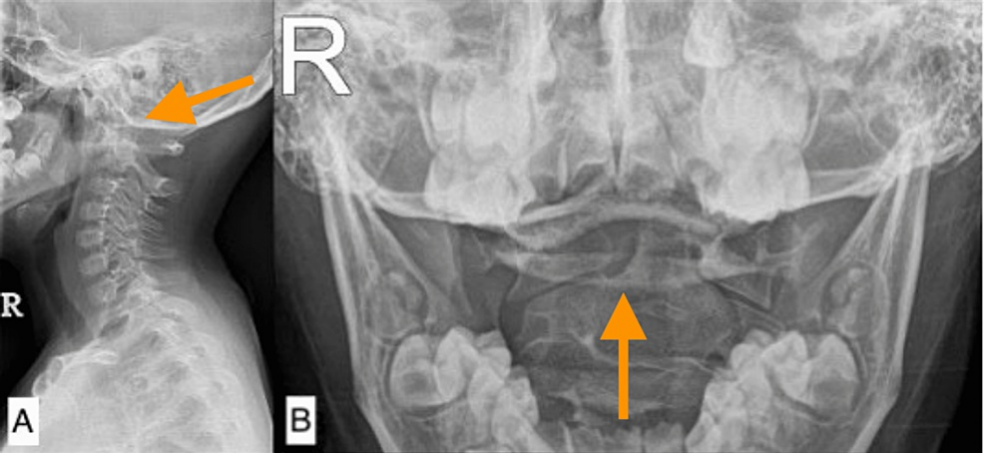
Pre-reduction cervical spine X-ray showing asymmetrical odontoid lateral interval and rotation: (A) lateral view and (B) open mouth view (arrows).
